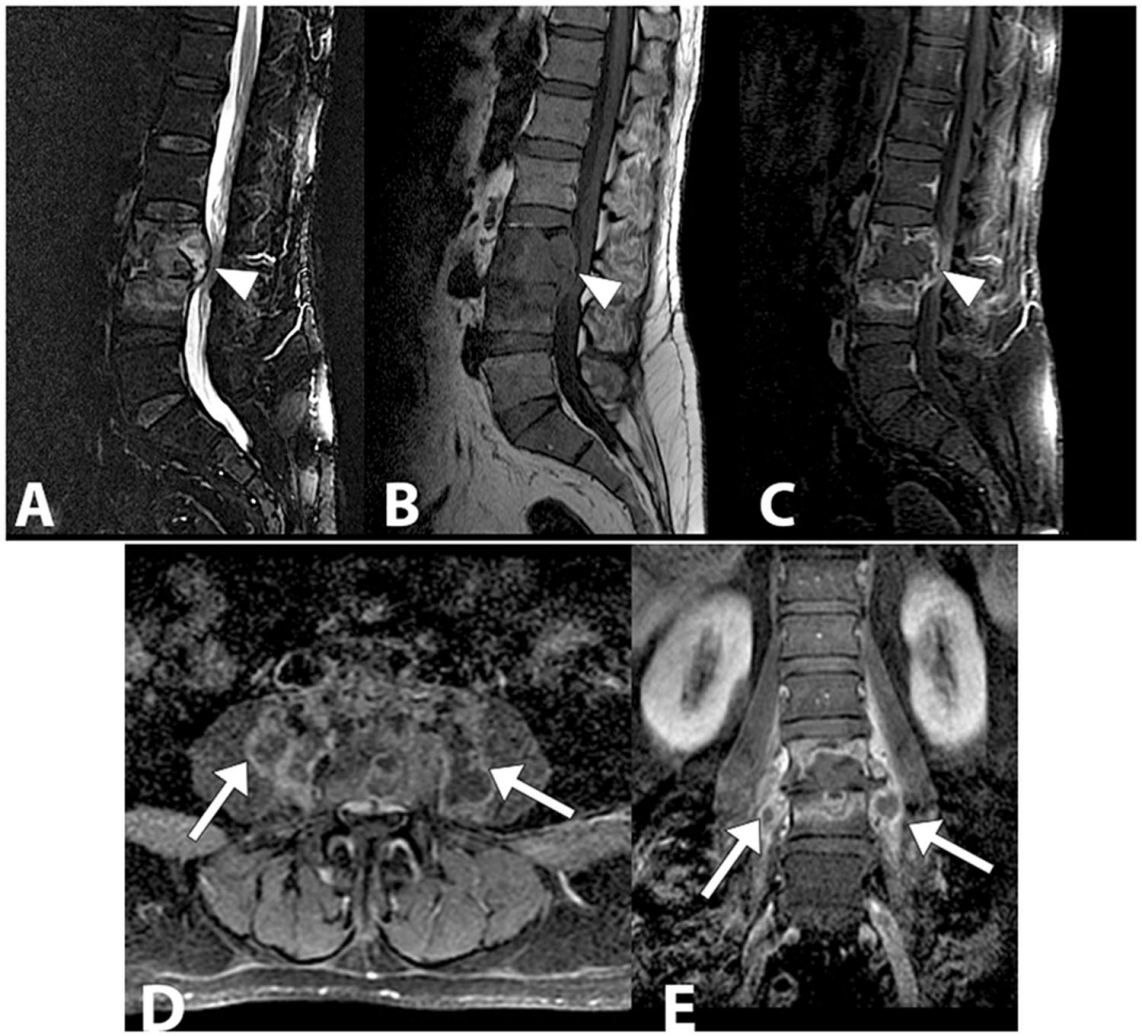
Lumbar tubercular spondylodiscitis. Sagittal STIR (a), sagittal T1 without contrast (b), sagittal T1 with contrast (c) images demonstrating severe osteo-structural alteration of the L3 vertebral body with invasion of the epidural space, dural sac and spinal roots compression (arrowhead). Axial (d) and coronal (e) T1-weighted images with contrast show paravertebral abscesses affecting the psoas muscles bilaterally (arrows)
